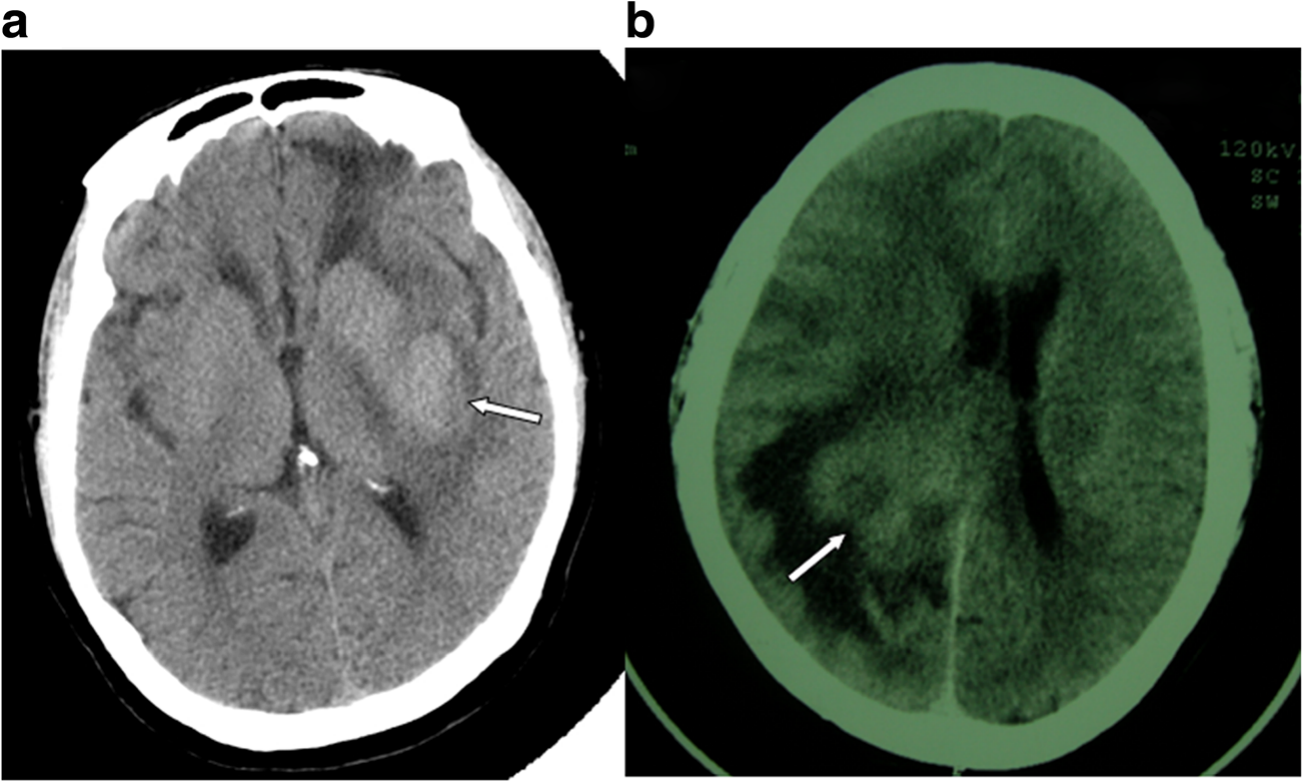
a CT features of primary central nervous system lymphoma (PCNSL) (arrow). b Obvious peritumoral edema (arrow)
